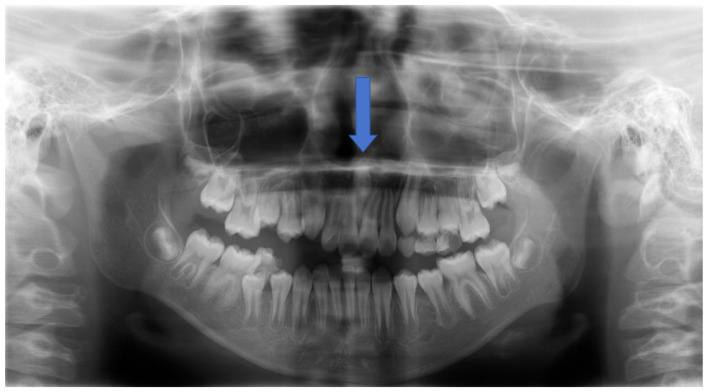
Panoramic radiograph demonstrating the inverted supernumerary tooth in the anterior maxilla (indicated by a blue arrow).
